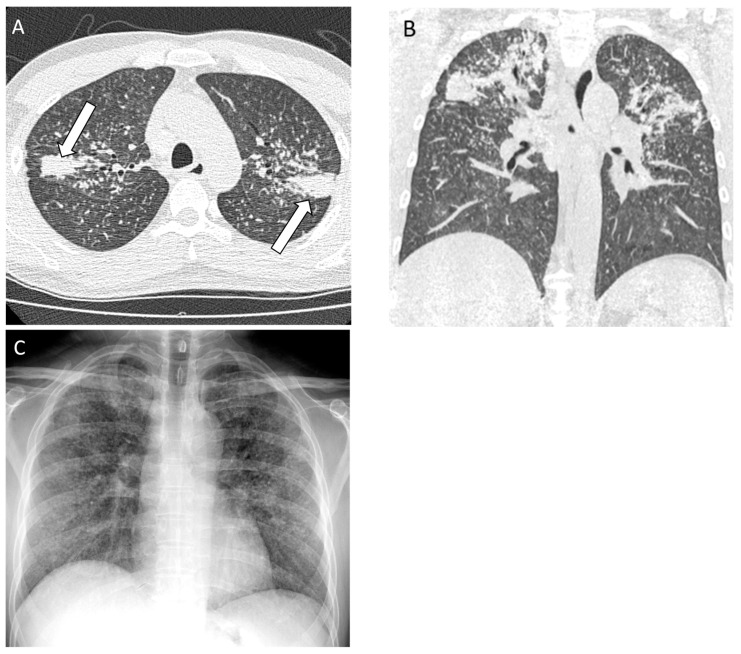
A 45-year-old non-smoking male, previously a stone mason and countertop stone cutter, diagnosed with complicated silicosis and progressive massive fibrosis. (A) Axial computed tomography image shows conglomerate bilateral upper zone masses with associated architectural distortion consistent with progressive massive fibrosis (white arrows); (B) coronal image demonstrates the upper lung zone predominance; (C) chest radiograph demonstrates profuse small circumscribed pulmonary nodules with an upper lung zone predominance.
